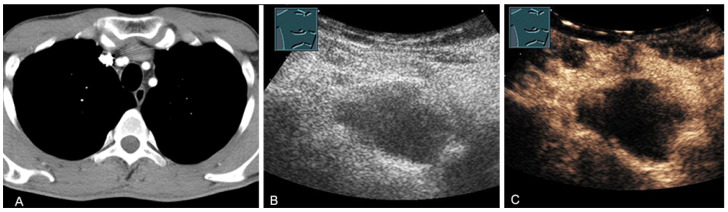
A cystic mediastinal mass on CT (provided by Prof. Dr. Andreas H. Mahnken, Marburg, Germany) (A), B-mode US (B), and CEUS (C), surgically confirmed as a mediastinally located bronchogenic cyst.
